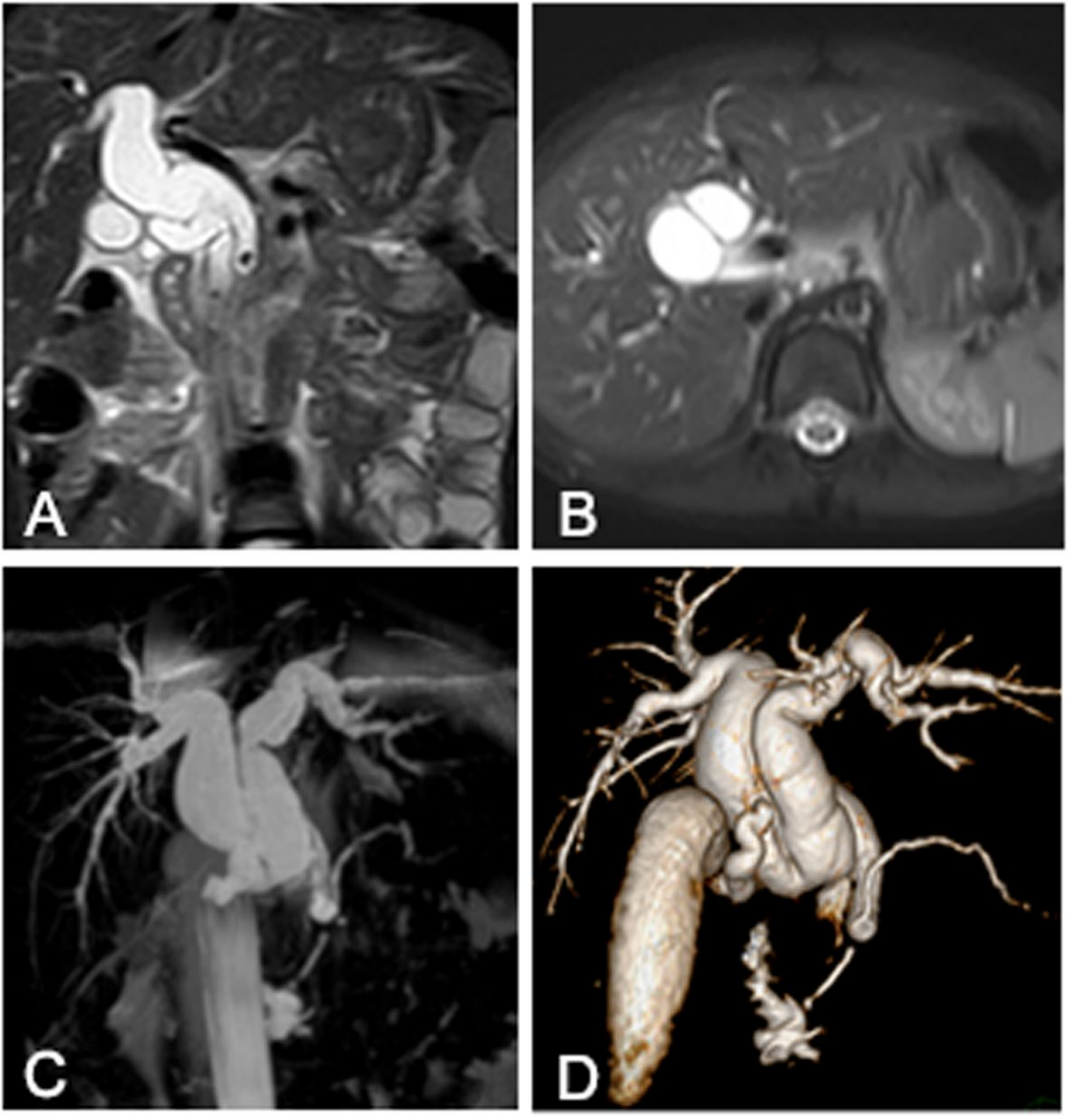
Preoperative MRCP showed the double common bile duct. A and B coronal and axial magnetic resonance T2-weighted image showed the low signal septum in the dilated common bile duct which divided the extrahepatic bile duct into two parts, which connected to the left and the right hepatic duct respectively. C and D coronal maximum intensity projection magnetic resonance image and volume rendering image showed the dilated and duplicated common bile duct
